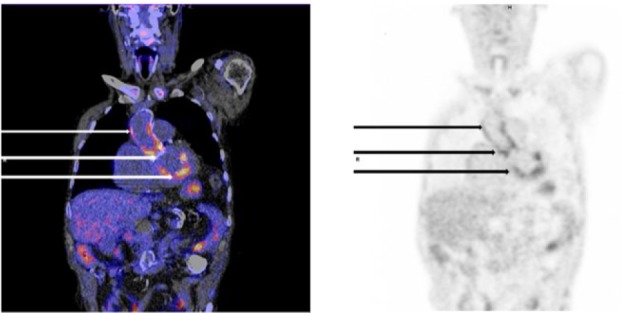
^18^F-FDG PET scan and CT scan Coronal view of the ^18^F-FDG PET in fusion with low dose CT (left) and only ^18^FDG PET (right) showing activity at the proximal ascending aorta (upper arrows), aortic root (middle arrows) and inferior wall of the heart (lower arrows).
